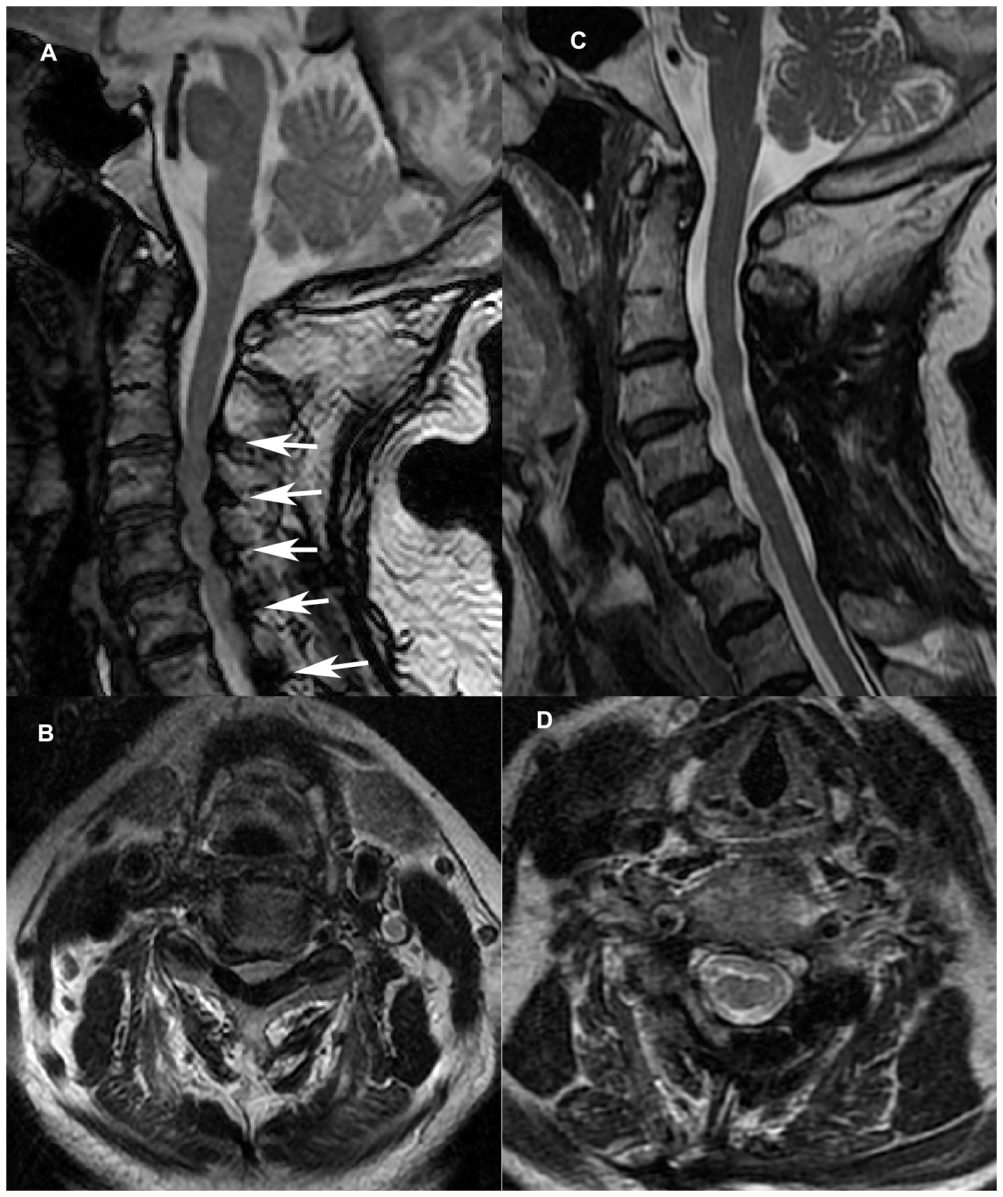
Pre- and postoperative MRI of a 57-year-old man with multilevel cervical canal stenosis. (A) Preoperative sagittal and (B) axial views of this MRI show the cervical spinal cord compressed by degenerative disk herniation at C3–4, C4–5 and C5–6 with five-level hypertrophic ligamentum flavum (arrows). (C) Postoperative sagittal and (D) axial MRI views 1 year after surgery demonstrate satisfactory decompression of the spinal cord. The dural sac has expanded significantly, and no restenosis is observed. MRI, magnetic resonance imaging.
